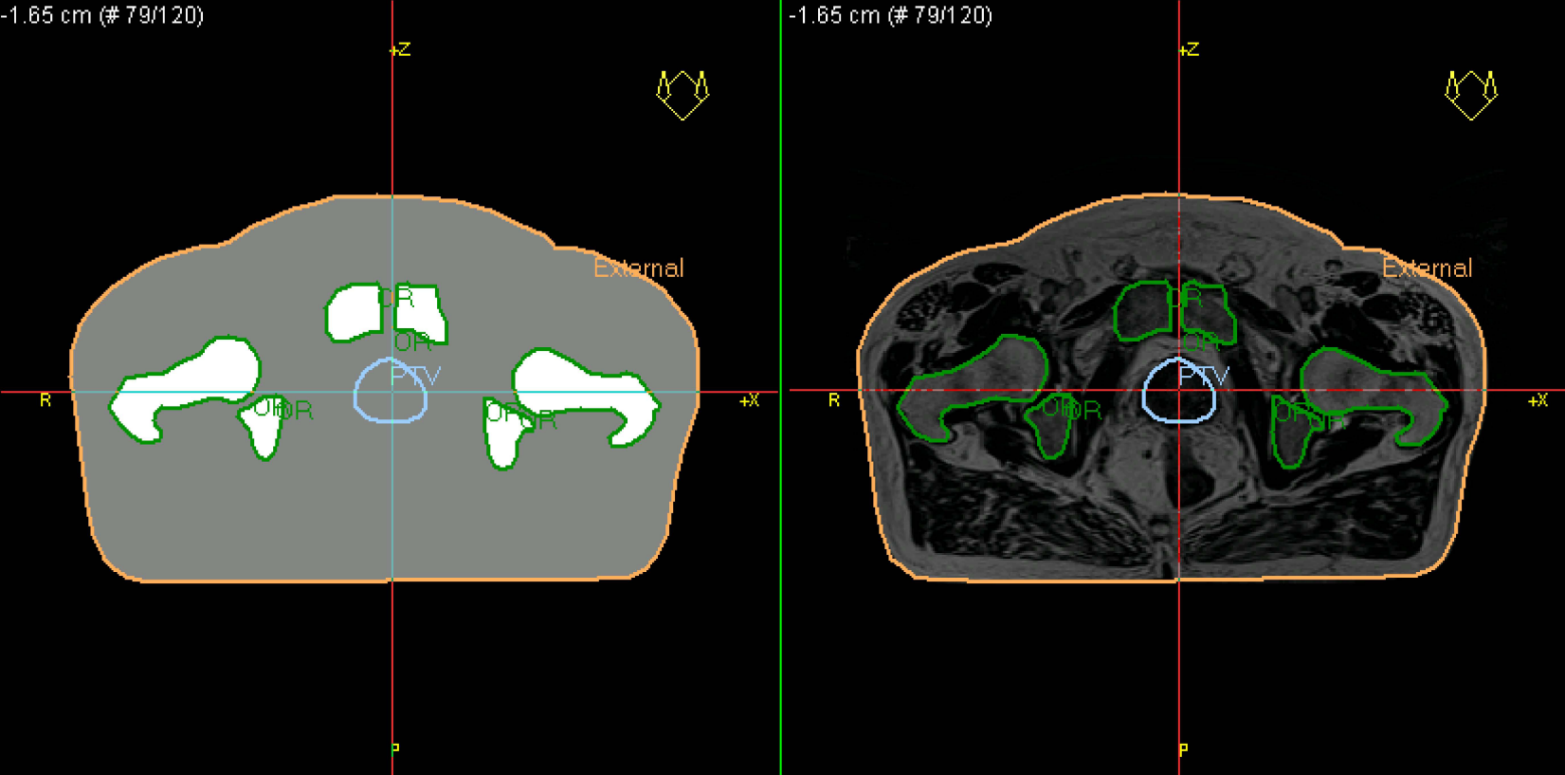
Synthetic CT and MR image. The synthetic CT with assigned mass densities (left) and the MR image on which it was based (right).
